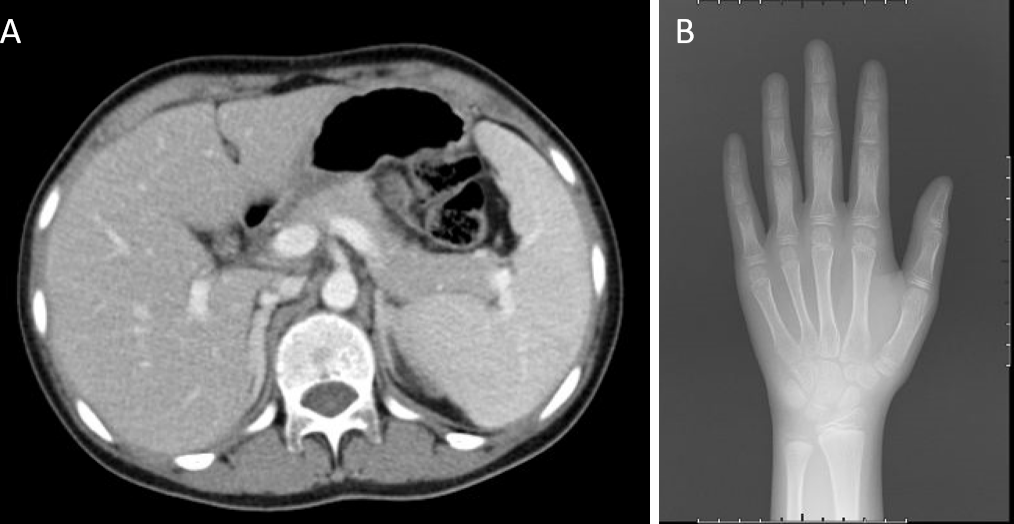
Imaging examinations. (A) An abdominal CT scan revealed no apparent hyperplasia in the bilateral adrenal gland. (B) X-ray showed her bone age was 8 years old.
